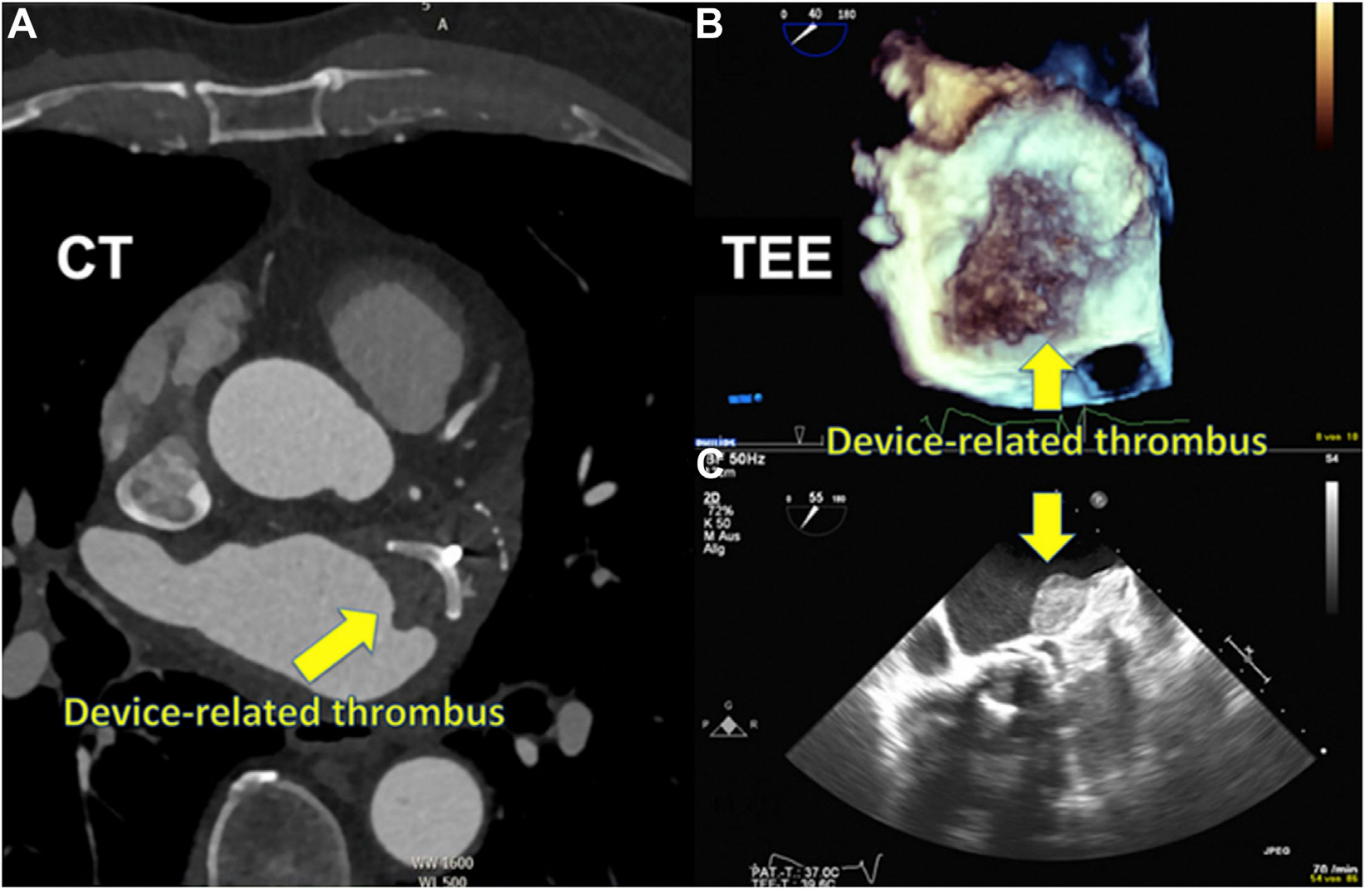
Device-related thrombosis (DRT) detected during imaging follow-up. (A) DRT detected using cardiac computer tomography in a 75-year-old patient 590 days after left atrial appendage closure. (B, C) DRT in 3- and 2-dimensional transesophageal echocardiography in an 87-year-old patient 226 days after left atrial appendage closure. CT, computed tomography; TEE, transesophageal echocardiography. Reproduced from Sedaghat et al,^71^ 2021.
